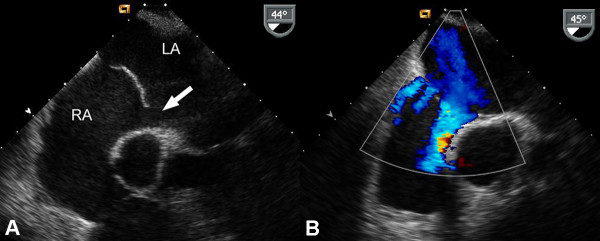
Aneurysmal atrial septal defect. (A) Transesophageal echocardiogram showing the left atrium (LA) and right atrium (RA). The atrial septal aneurysm can be seen bulging into the left atrium, and the ASD is shown (arrow). (B) Color flow Doppler across the atrial septum demonstrates multiple fenestrations with three distinct jets seen crossing the septum.
